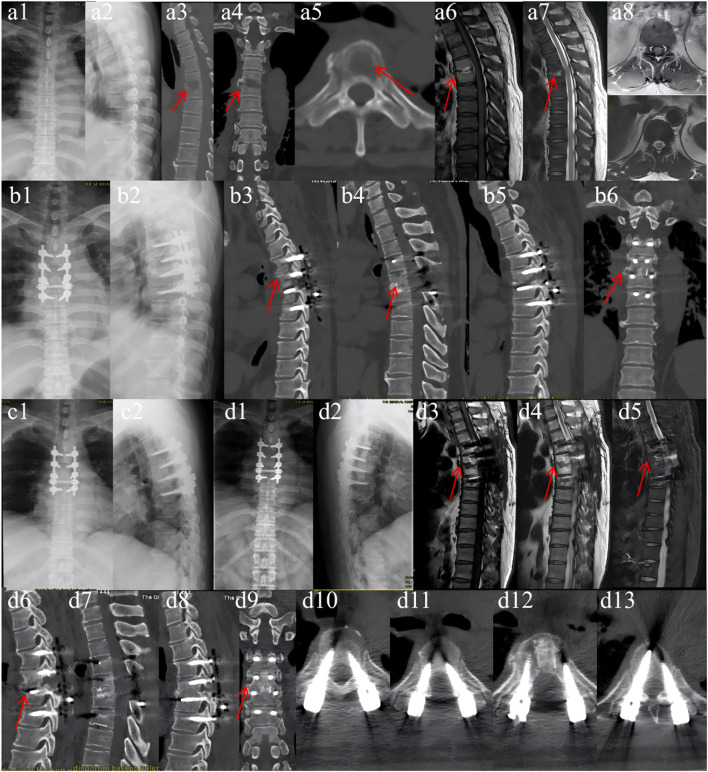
Preoperative and postoperative follow-up imaging of a patient with T5–T6 *Klebsiella pneumoniae* infection treated with direct pedicle screw fixation involving the infected vertebrae. Preoperative imaging: (a1, a2) Anteroposterior and lateral radiographs. (a3–a5) Sagittal and axial CT images revealing severe bone destruction at the T5–T6 vertebral interface and a cavity in the T6 vertebral body. (a6–a8) Sagittal and axial MRI demonstrating bone marrow edema in the T5–T6 vertebral bodies, along with intervertebral space and epidural abscesses. Postoperative imaging at 3 days: (b1–b6) X-ray and CT images. Postoperative imaging at 3 months: (c1, c2) Anteroposterior and lateral radiographs. Postoperative imaging at 9 months: (d1, d2) Anteroposterior and lateral radiographs. (d3–d5) Sagittal MRI demonstrating no recurrence of abscess. (d6–d13) Sagittal and axial CT images confirming that the internal fixation is in good position.
